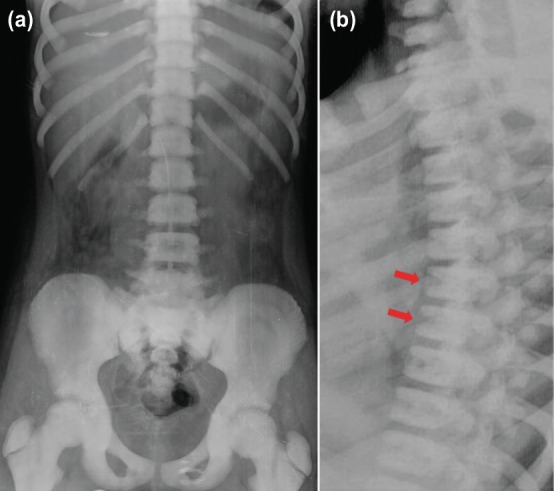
(a) Anteroposterior and (b) lateral spinal column radiographs. The red arrows indicate typical sandwich vertebrae.
